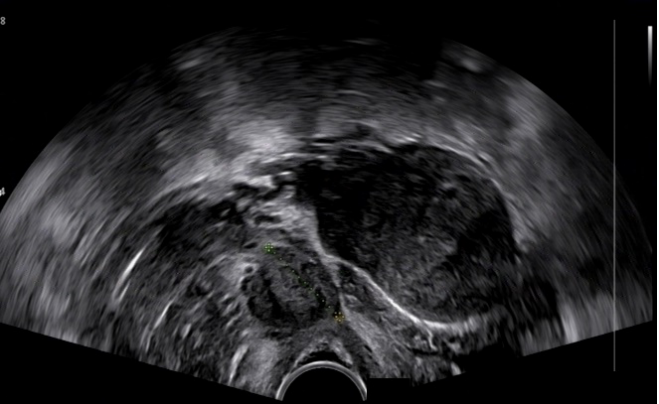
(# Case 2). Ultrasound scan depicting the 4cm leiomyoma at the anterior vaginal wall (transverse plane).
